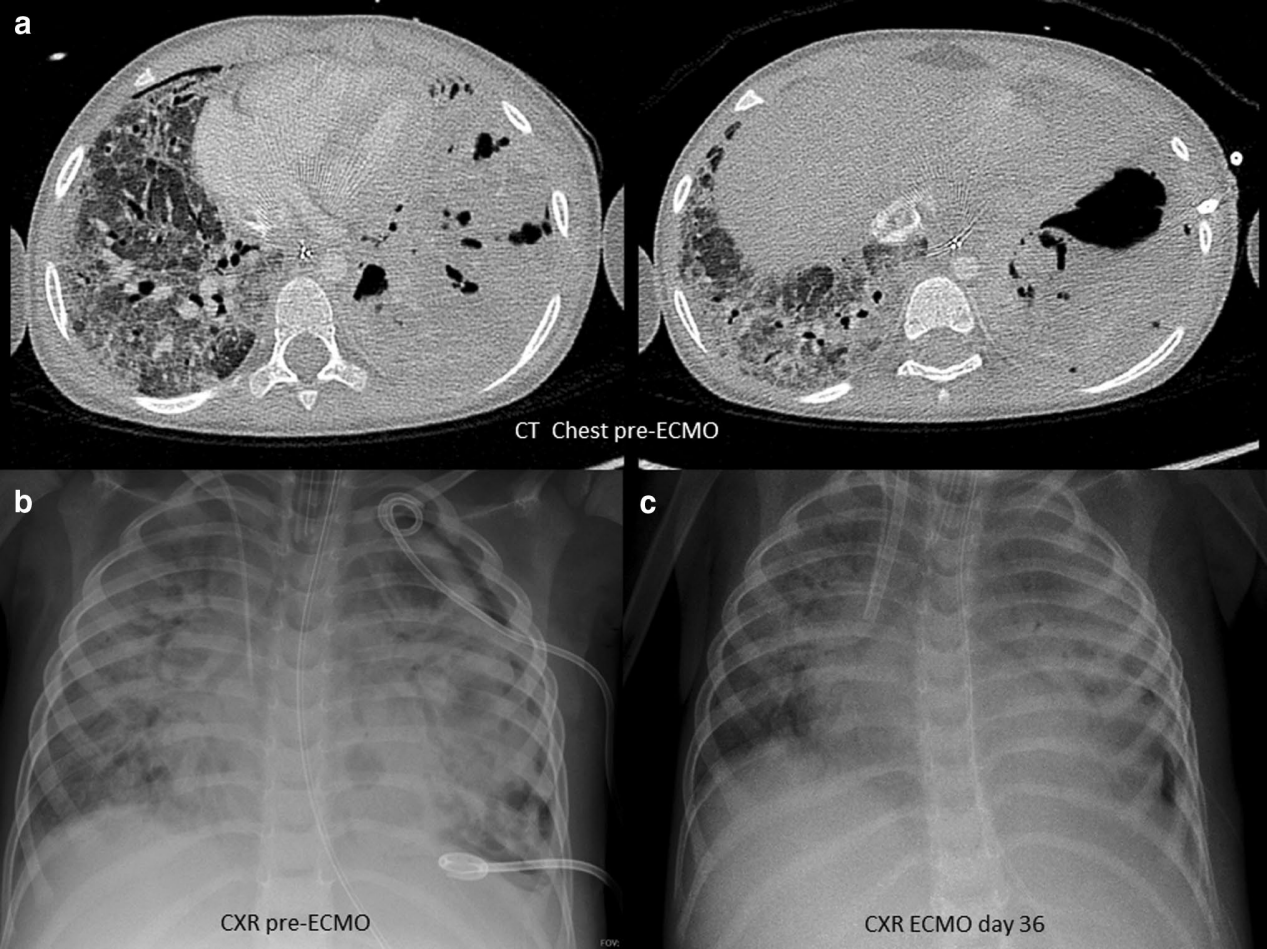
Chest computer tomography before ECMO and chest X-ray before and during ECMO
